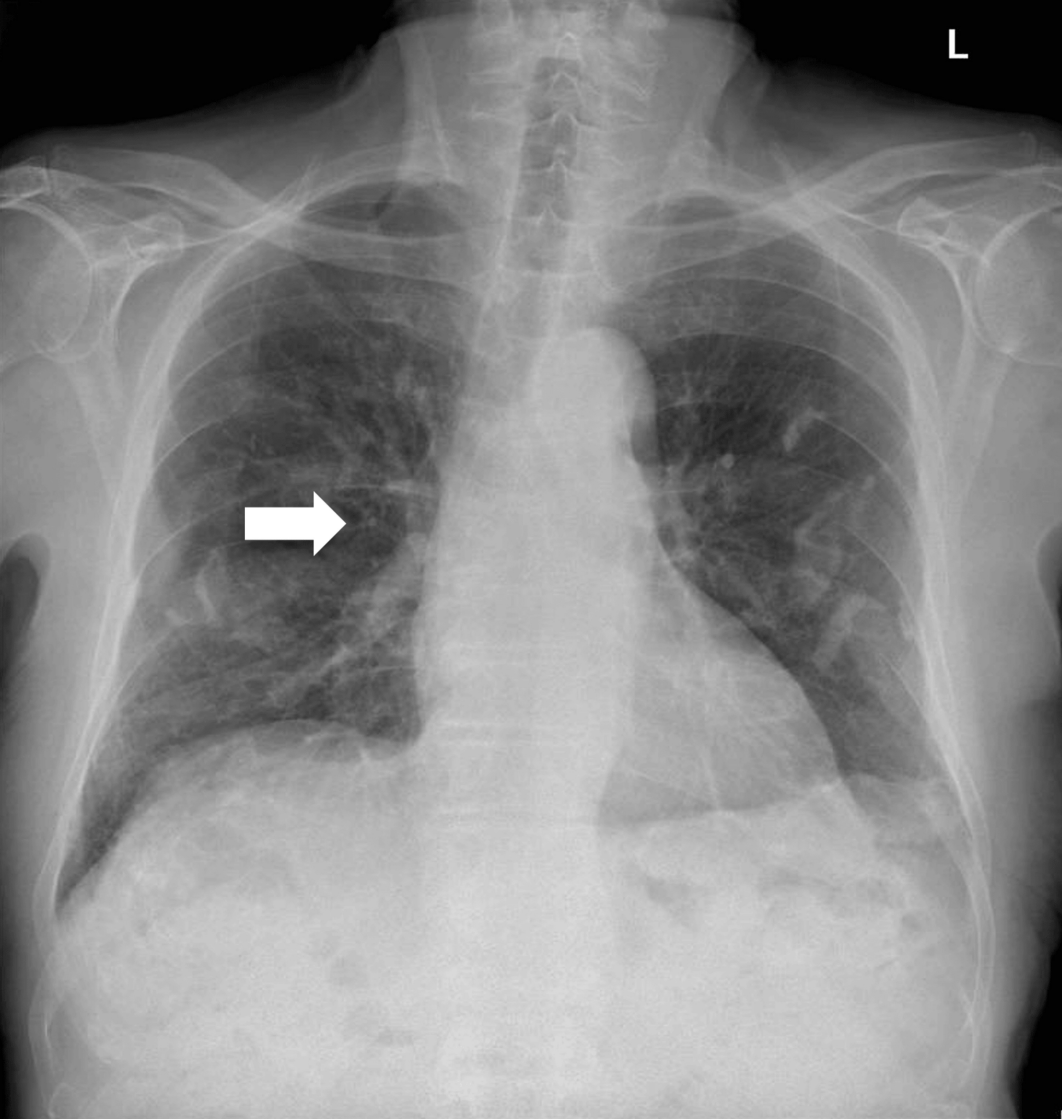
Chest X-ray on admission White arrows highlight longstanding bilateral pleural plaques. The arrowhead indicates a 6 mm right hilar nodule consistent with known metastatic disease.
